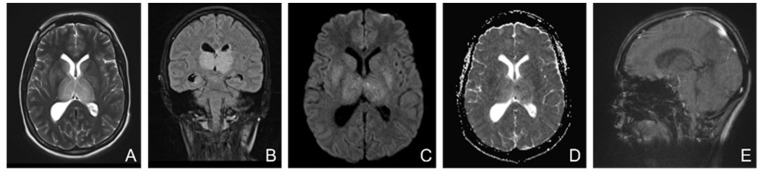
MRI without contrast enhancement ((A)—T2 axial, (B)—FLAIR coronal, (C)—DWI axial, (D)—ADC axial, (E)—venous TOF sagittal) shows hyperintensities of the thalamic nuclei on T2, FLAIR and DWI, with areas of restricted diffusion included and no flow in Galen’s vein, internal cerebral veins, straight sinus, and inferior sagittal sinus.
